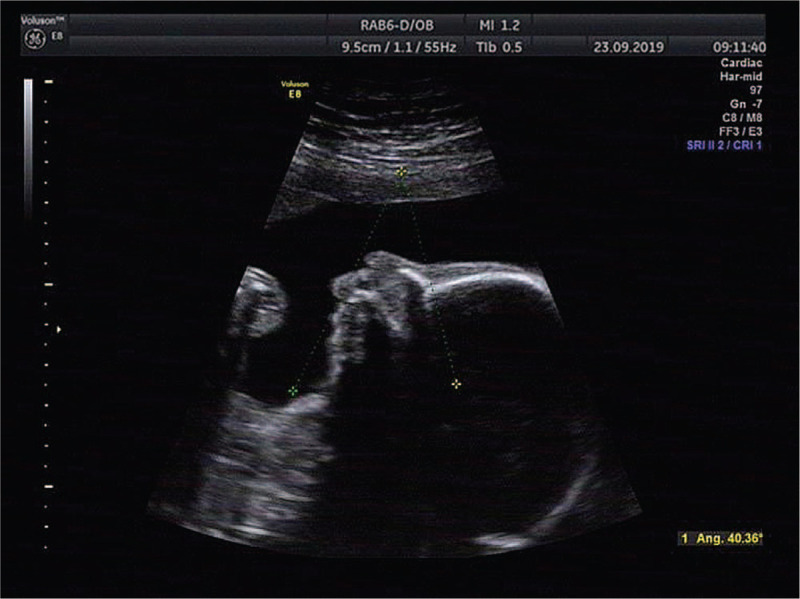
The fetal ultrasound imaging showed micrognathia.
